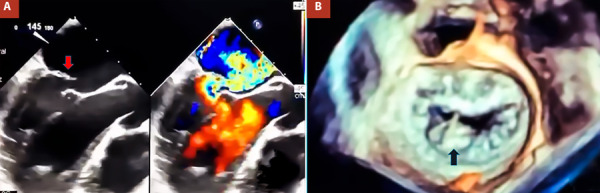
A. Pre-operative transesophageal echocardiography showing P2 flail (145o view) (red arrow). B. 3D mitral valve reconstruction showing P2 flail (black arrow).
